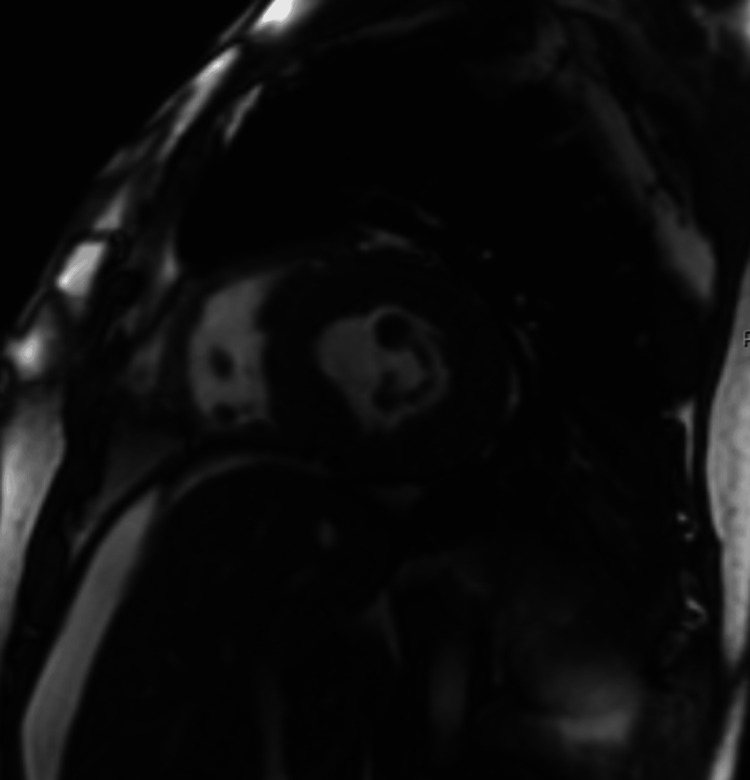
Cardiac MRI with short-axis imaging demonstrating hypertrophy of the left ventricular wall.
